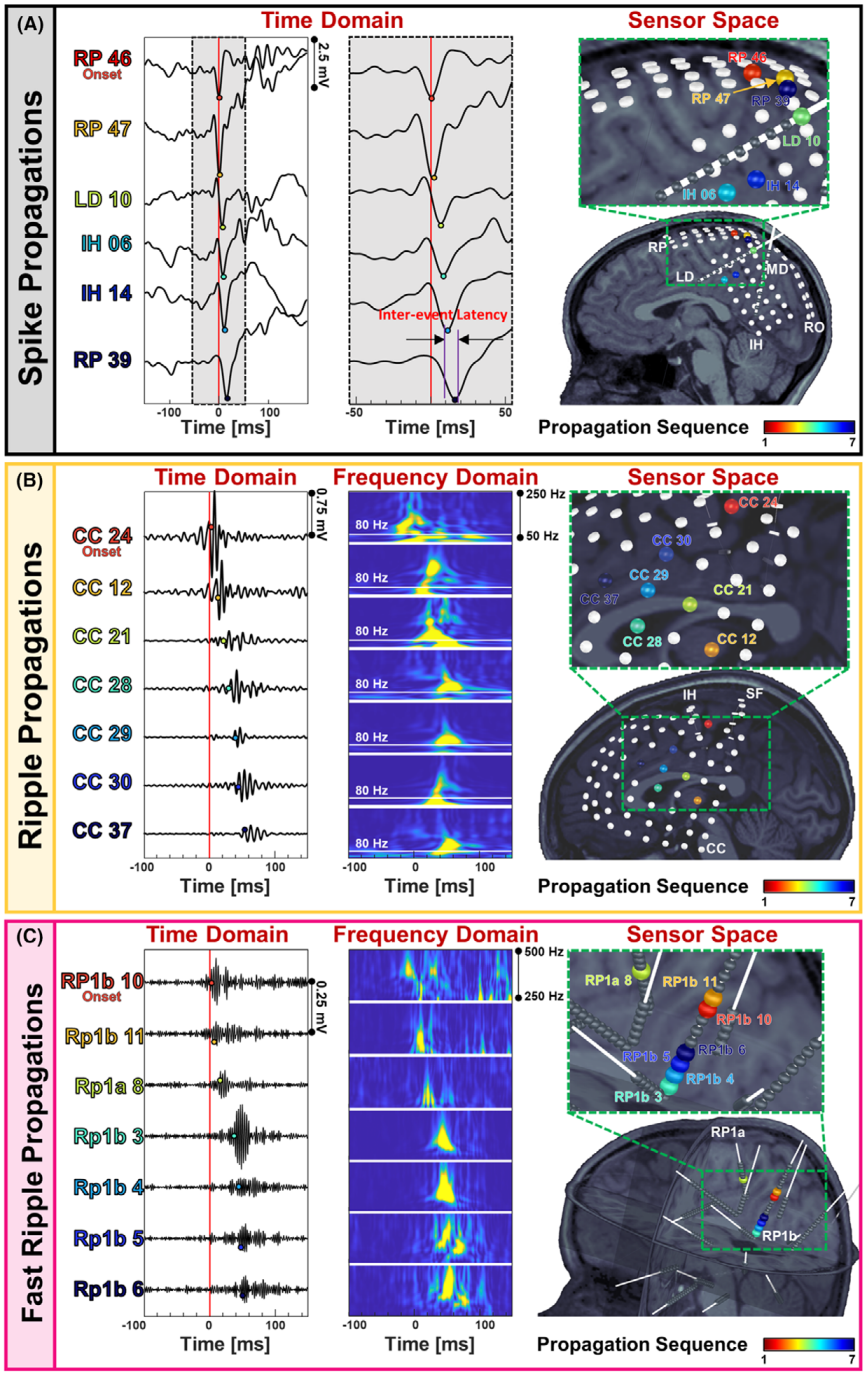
Sequences of spike, ripple, and fast ripple propagations. (A) Spike propagation in time domain (ms) across depth electrodes implanted in a 9‐year‐old female patient with drug‐resistant epilepsy (DRE) (patient #8). The first event in propagation is considered as the onset event, followed by the next events. The time window between two successive events (i.e., *inter‐event interval*) in a spike propagation was set to 10 ms. Electrodes that participate in propagation are color‐coded according to their order in propagation. The propagation sequence is shown color‐coded on electrodes co‐registered on patient's MRI, with the onset electrode colored in red and the last electrode in the propagation colored in blue. (B) Ripple propagation in time domain (ms) across subdural electrodes implanted on the cortex of a 11‐year‐old male patient with DRE (patient #1). The *inter‐event interval* for ripple propagations was set to 30 ms. Time‐frequency plots of each of the events in propagation are also displayed (50–250 Hz); the 80 Hz frequency is indicated to define the lower boundaries of the ripple frequency band. The sequence of this propagation is showed on patient's MRI. The electrodes that participate in the propagation are colored according to the order they appear in propagation. (C) Fast ripple propagation in time domain (ms) across depth electrodes implanted in a 6‐year‐old female patient (patient #23). The *inter‐event interval* for fast ripple propagations was set to 15 ms. The time‐frequency plot of each of the events in the propagation is plotted (250–500 Hz). The propagation sequence is shown color‐coded on electrodes co‐registered on patient's MRI. The electrodes that participate in the propagation are colored according to the order they appear in the propagation.
